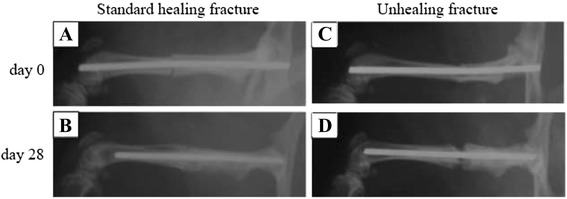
Radiographs of the standard healing fractures (a and b) and unhealing fractures (c and d) obtained immediately post-fracture (a and c) and at post-fracture day 28 (b and d)
